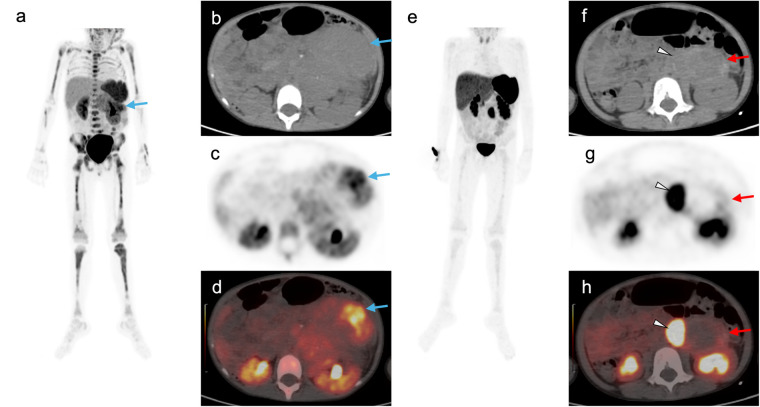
(a) maximum intensity projection (MIP); (b) axial CT; (c) axial PET; (d) fused PET/CT; (e) MIP; (f) axial CT; (g) axial PET; and (h) fused PET/CT.
